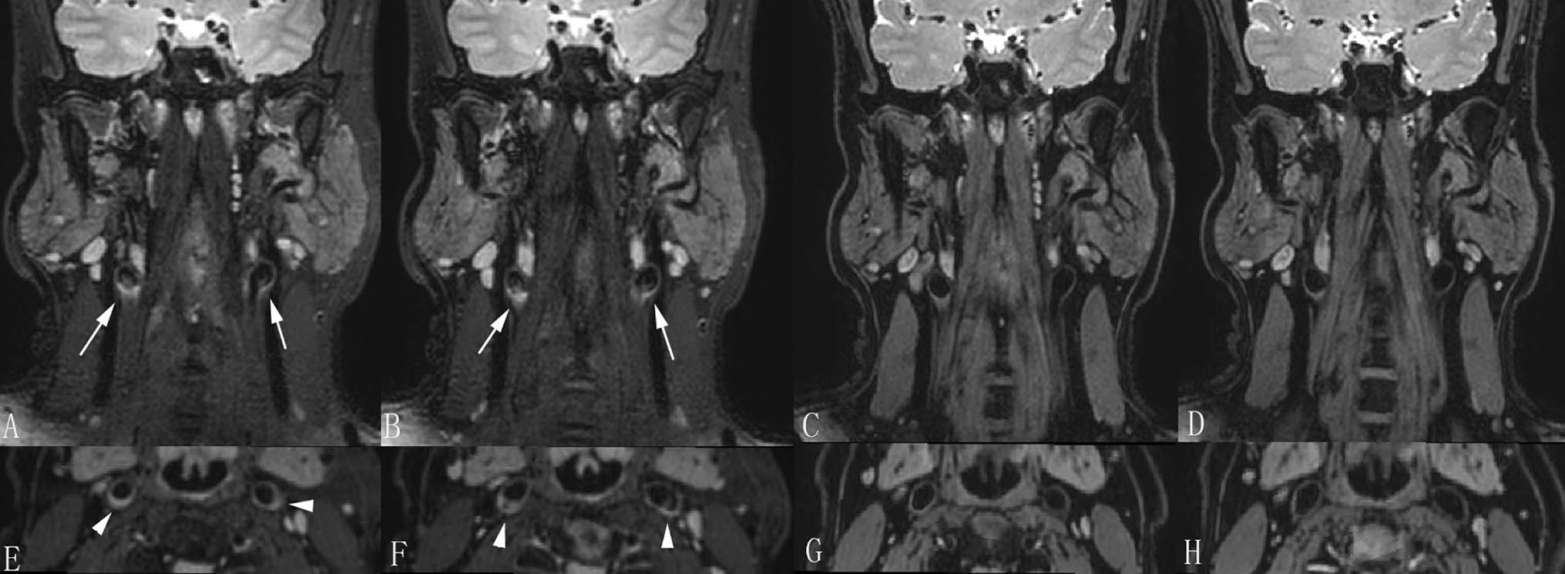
Examples of residual flow artifacts at the carotid bifurcation. (A,B,E,F) Presence of residual flow artifacts at the carotid bifurcation (arrows on the coronal plane and arrowheads on the reconstructed transverse plane) presented on 3D T2-weighted fast field echo (3D_T2_SPACE). Note that the artifacts may lead to overdiagnosis of plaques. (C,D,G,H) No residual flow artifacts at the carotid bifurcation were presented on 3D_T2_FFE (Same subject).
